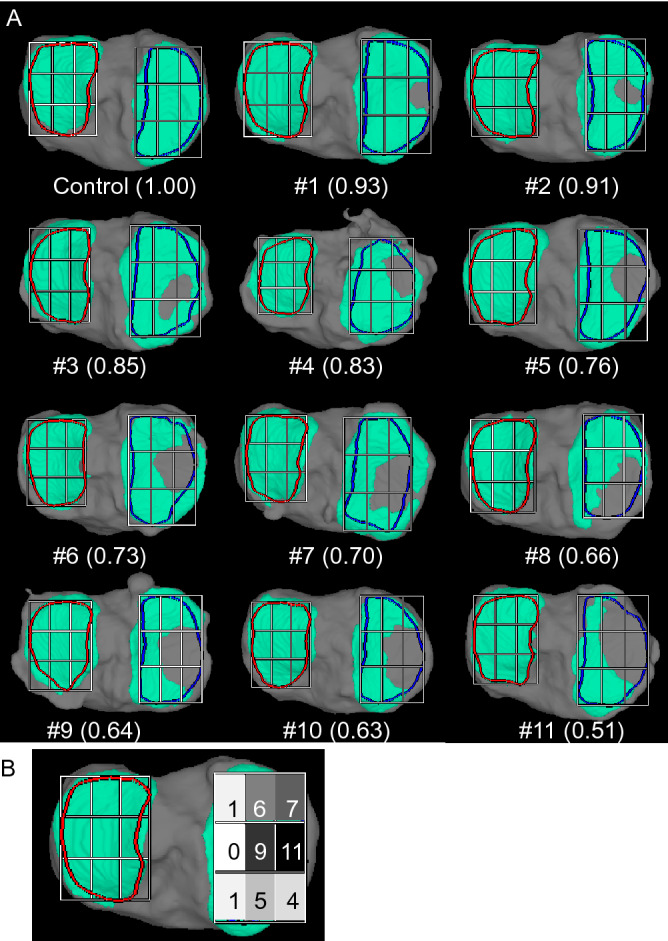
Location of defects in the medial tibial cartilage. (A) Cartilage area of the tibia determined by 3D magnetic resonance imaging (MRI). The tibial cartilage is shown in green, and the region of interest (ROI) of the medial tibial cartilage is shown enclosed within the blue line in the fully automated 3D-constructed MRI image. The cartilage area ratio is defined as the ratio of the cartilage area to the ROI area. The cartilage area ratio at the medial tibia is also shown, and the images are ordered from the highest to the lowest ratios. The ROI of the medial tibial cartilage was divided into 9 subregions, with 3 equal vertical and 3 equal horizontal lines. (B) Number of knees with cartilage defects in each subregion among 11 knees. The thickness of the cartilage is indicated by color mapping, where white indicates a thickness ≥ 2 mm and the progression from yellow to red indicates thinning of the cartilage. The tibia bone is shown in gray.
